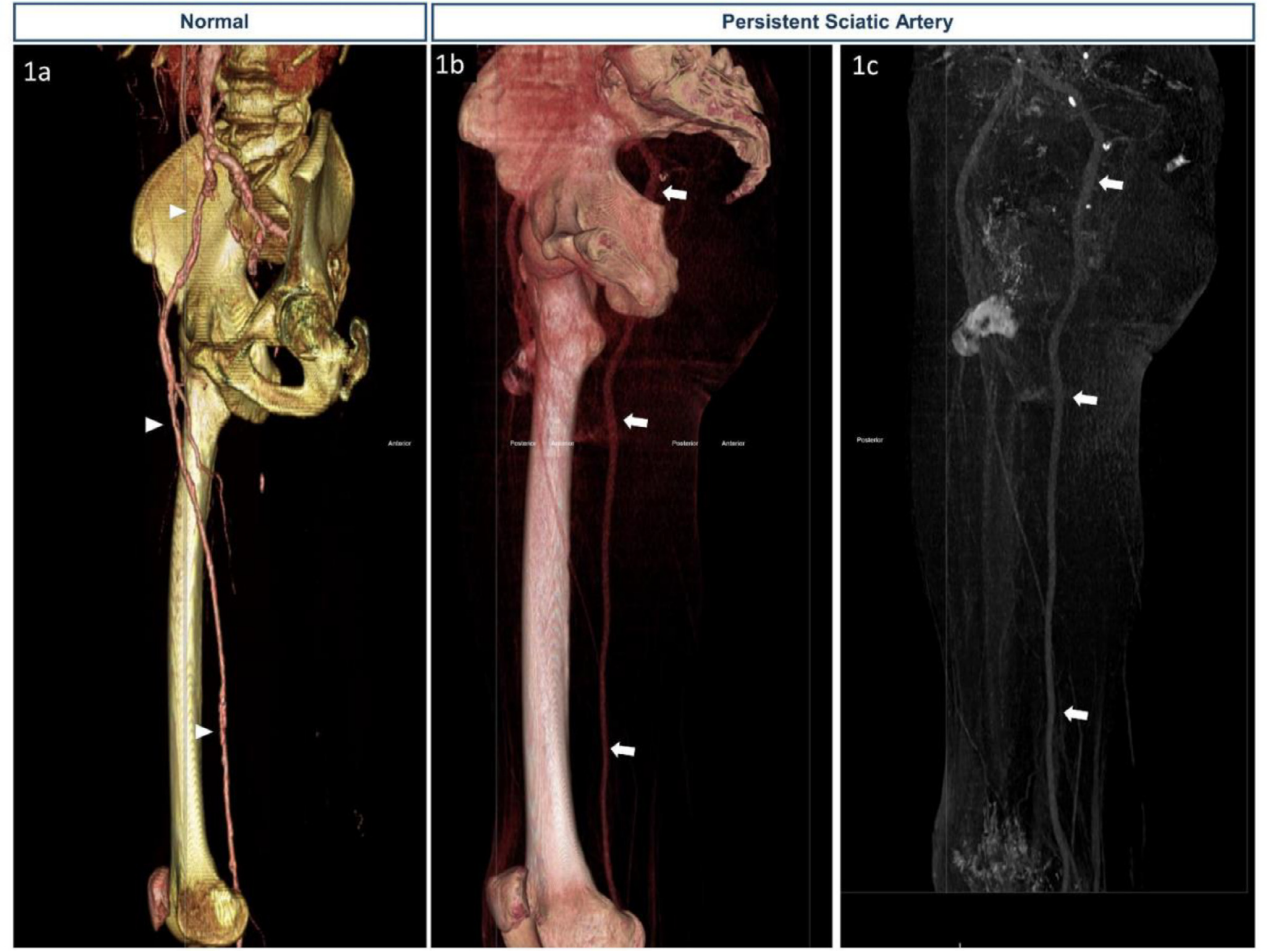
A 43-year-old male presented with a right leg ulcer. Findings: (1A) is a CTA 3D reconstruction of the right lower extremity demonstrating the normal anatomy of the common femoral artery, superficial femoral artery, and its distal continuation as the popliteal artery provided for comparison (arrow head). (1B) and (1C) 3D reconstruction of CT of the right femur with contrast demonstrating persistent sciatic artery with hypoplastic superficial femoral artery.
